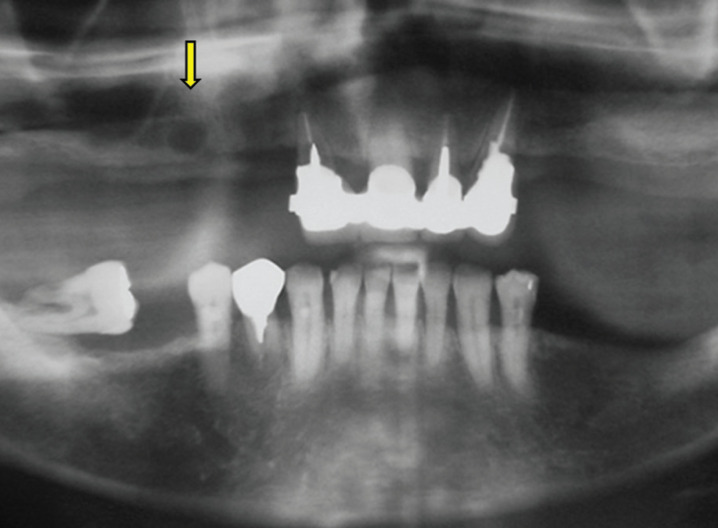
Panoramic radiograph showing an unilocular radiolucency in the right maxilla (arrow).
